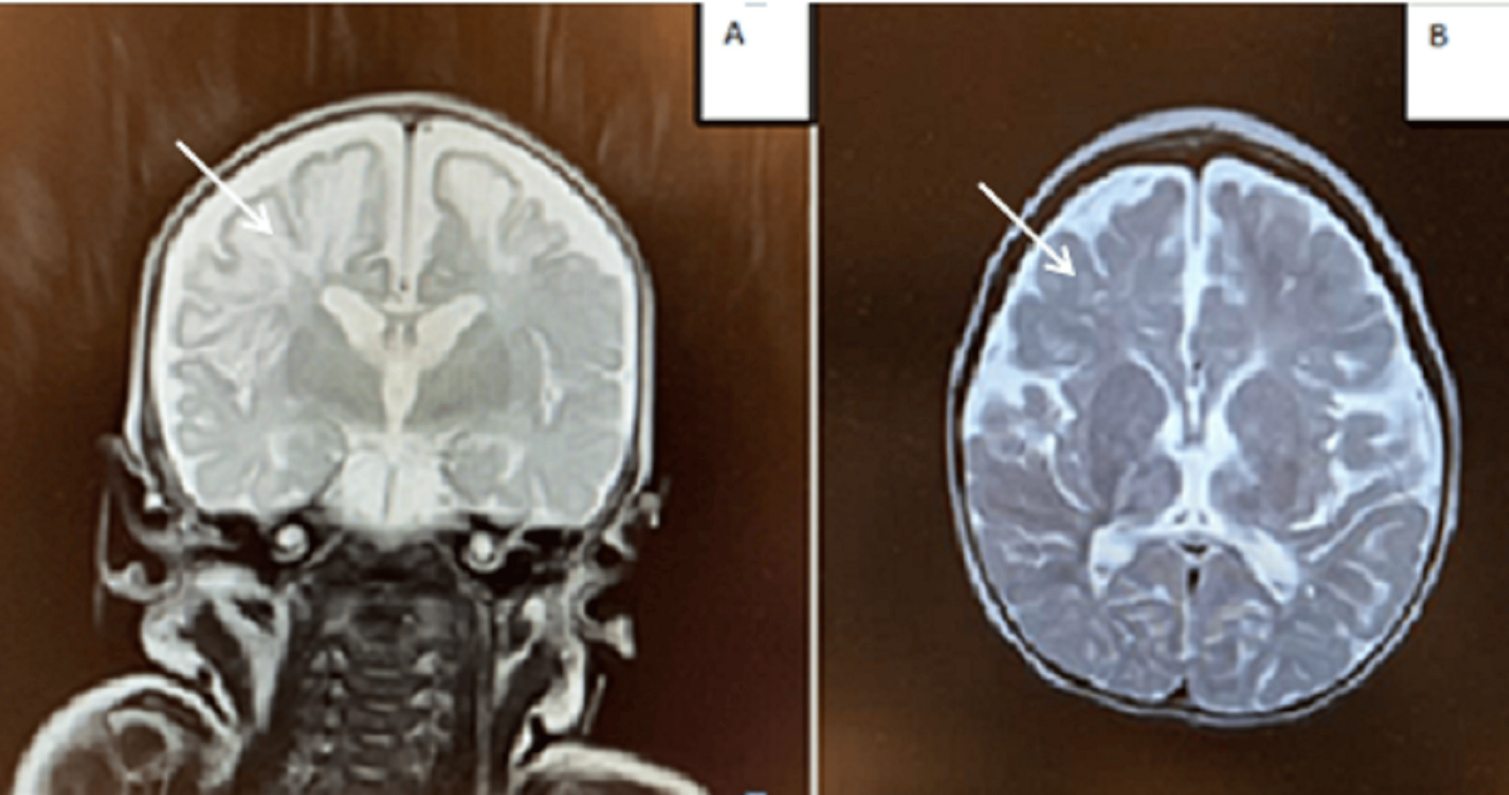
Brain MRI in T2 sequence. (A) Coronal section at one week of age showing diffuse hyperintensity of the white matter (arrow). (B) Axial section at 25 months of age showing persistent diffuse hyperintensity of the white matter with very limited myelination (arrow).
